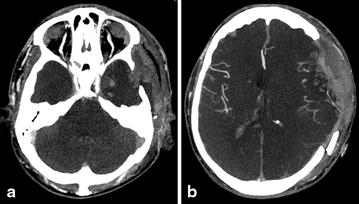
CTA axial views of a clinically brain-dead patient with a craniectomy and no opacification of the SPV (a) despite persistent enhancement of the bilateral C-MCA (b)
